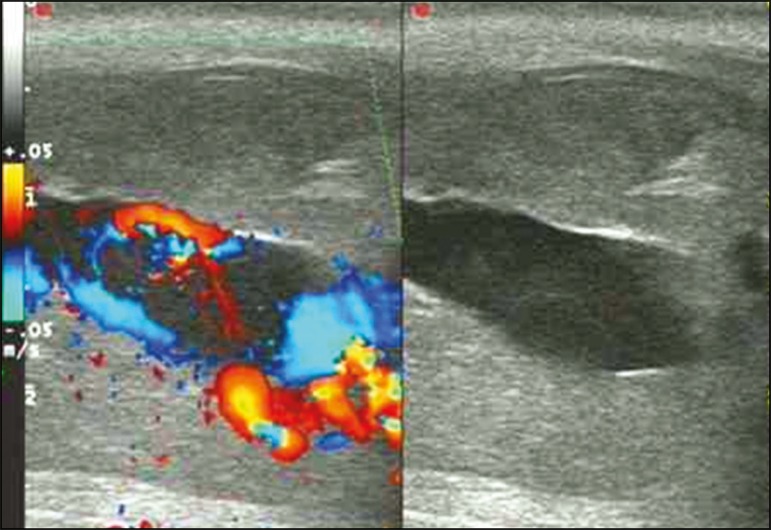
Color Doppler ultrasound demonstrating a hypoechoic collection that
